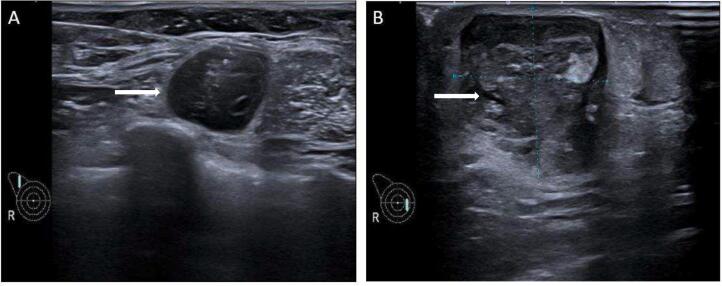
Ultrasound showed two lobulated, hypoechoic, solid lesions (white arrows) in right breast. (A) Near axillary tail (B) Lower inner quadrant.
